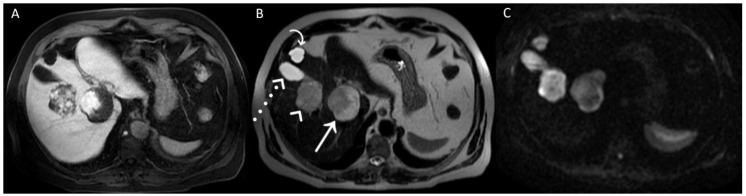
A posteriori, a perfect correspondence between the contrast agent globular enhancement on Axial T1-weighted fat saturated images in delayed phase (A) and the relatively hypointense areas on post-contrast T2WI (B) and DWI (C) was evident. Furthermore, the largest and deepest lesion showed a relatively hypointense area on DWI, larger than that on T2WI, due to later acquisition of DWI and progressive centripetal contrast filling of the lesion. On T2WI (B), we can also appreciate the comparison between the signal hyperintensity of different structures: cavernous hemangiomas (arrow and arrowhead); simple cyst (curved arrow), and gallbladder’s fundus (dotted arrow). T2WI and DWI are usually acquired after the acquisition of vascular phases in the dead time until the acquisition of the hepatobiliary phase to shorten the time-consuming protocols since it was demonstrated that T2WI and DWI acquired after Gd-EOB-DTPA showed a comparable diagnostic capability to pre-contrast T2WI and DWI in the detection and characterization of hepatic tumors [1,2]. In conclusion, when liver lesions of unknown nature must be characterized, with a lack of previous radiological investigations, it could be useful to acquire pre-contrast T2WI and DWI to avoid diagnostic confusion, especially in non-tertiary centers.
